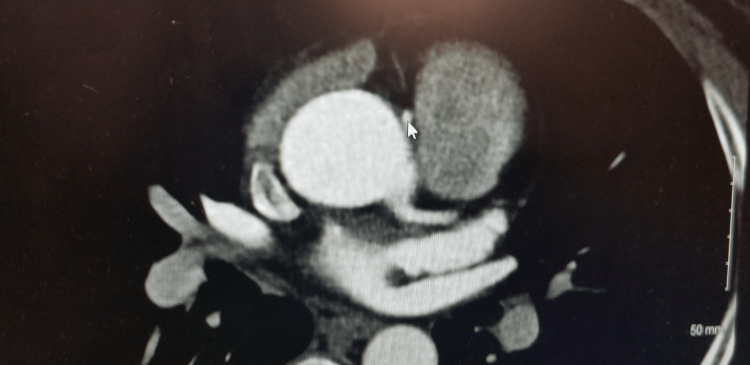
Interarterial course of the right coronary artery as seen on computed tomography angiogram.
